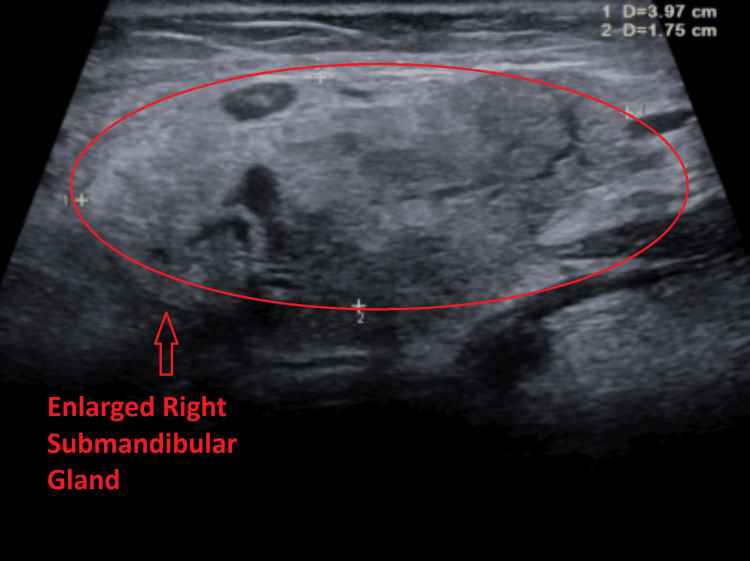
Ultrasound image of the transverse view of the right submandibular gland
